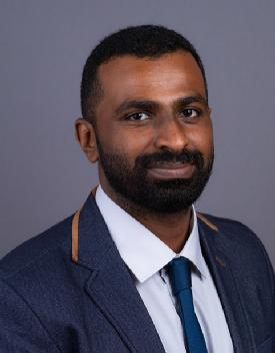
Abdalla Eltayeb Abdalla Abdelkader, MD, is an Assistant Professor in the Division of Cardiovascular Medicine, Department of Medicine, College of Medicine at the University of Florida. His clinical and research interests focus on echocardiography and multimodality cardiac imaging, with particular expertise in mitral valve prolapse and valvular heart disease. He is actively engaged in advancing quantitative imaging approaches to improve the assessment and management of complex valvular disorders.
